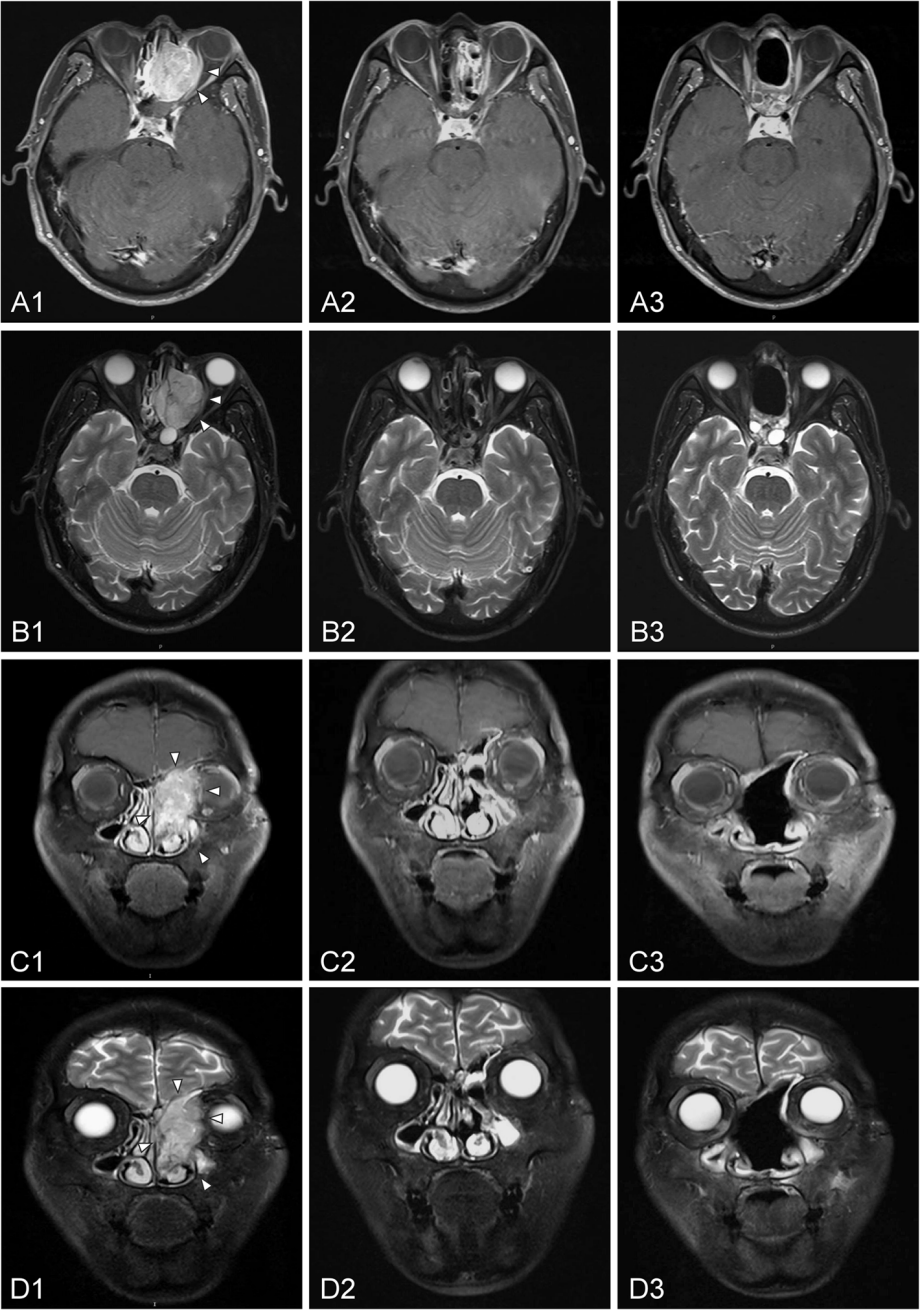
Comparison of MRI before and after treatment for a 43-year-old patient with ENB (stage of C) who received preoperative radiation plus surgery. (A) and (B) refer to axial MRI, and (C) and (D) refer to coronal MRI. 1, 2, and 3 refer to MRI before treatment, after preoperative radiation, and after surgery, respectively. MRI before treatment revealed a large mass on left nasal cavity and ethmoid sinus, which invaded the extraocular muscle of the left orbit (A1 arrowhead, B1 arrowhead). The tumor extended to left anterior skull base (C1 arrowhead, D1 arrowhead)
